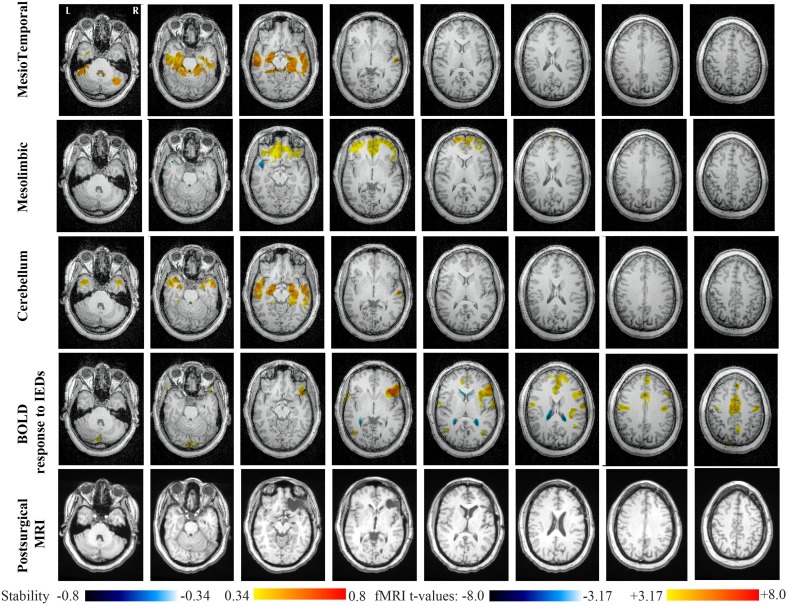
Evaluation of DANI on Patient 1 with right Orbito-Frontal epilepsy. Results are presenting most salient stability changes observed in *Cmap*_*n*_ followed by a t-map of the BOLD response to epileptic discharges and a postsurgical T1 MRI, all resampled in the native anatomical MRI space of the patient. Most salient stability changes observed for *Cmap*_*n*_ of Mesio-Temporal, Mesolimbic, and Cerebellum networks are presented. These three networks were also involved in significant interactions of modularity changes at *p* < 0.001. *Cmap*_*n*_ are presented between 0.34 and 0.8 (resp. −0.34 and −0.8) and BOLD *t*-map between 3.17 and 8.0 (resp. −3.17 and −8.0) using a yellow–red colormap (resp. white–blue colormap).
